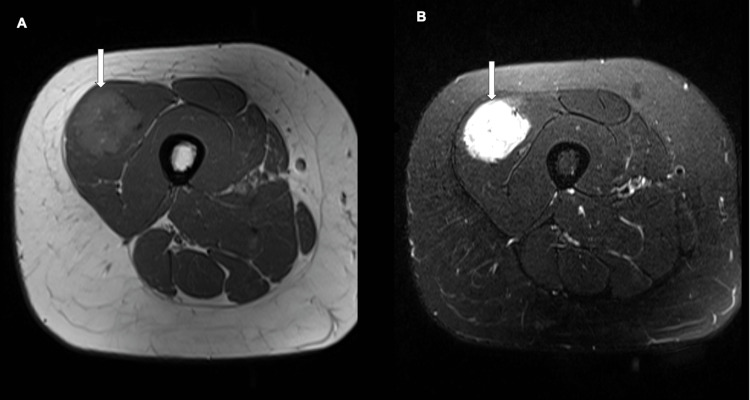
Axial MRI of the right thigh: (A) T1; (B) T2; arrow: pointing to the lesion of interest. MRI, magnetic resonance imaging
